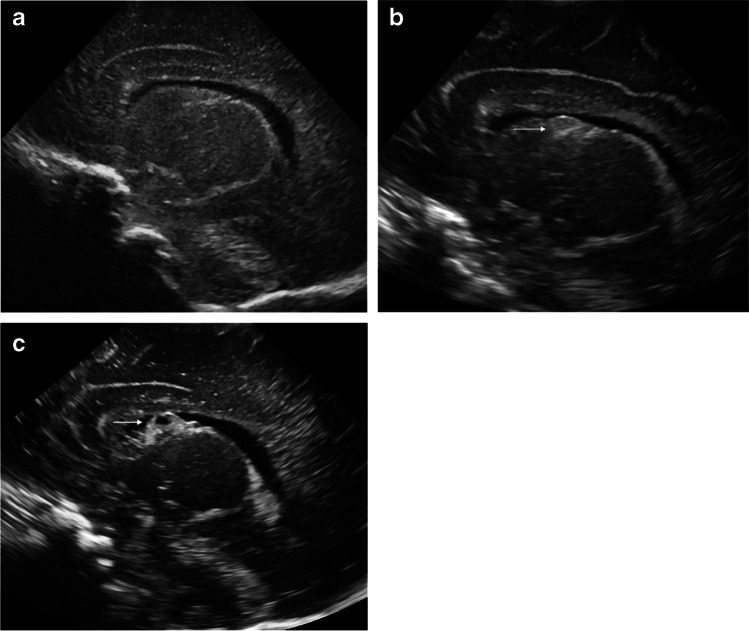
A sagittal image from a normal head ultrasound (US) at 7 days of life in a girl born at 31 weeks’ gestational age (a). A sagittal image from a follow-up US at 30 days shows new grade 1 germinal matrix hemorrhage (*arrow*) (b). A sagittal image from a subsequent US at 50 days shows expected cystic evolution of the germinal matrix hemorrhage (*arrow*) (c)
